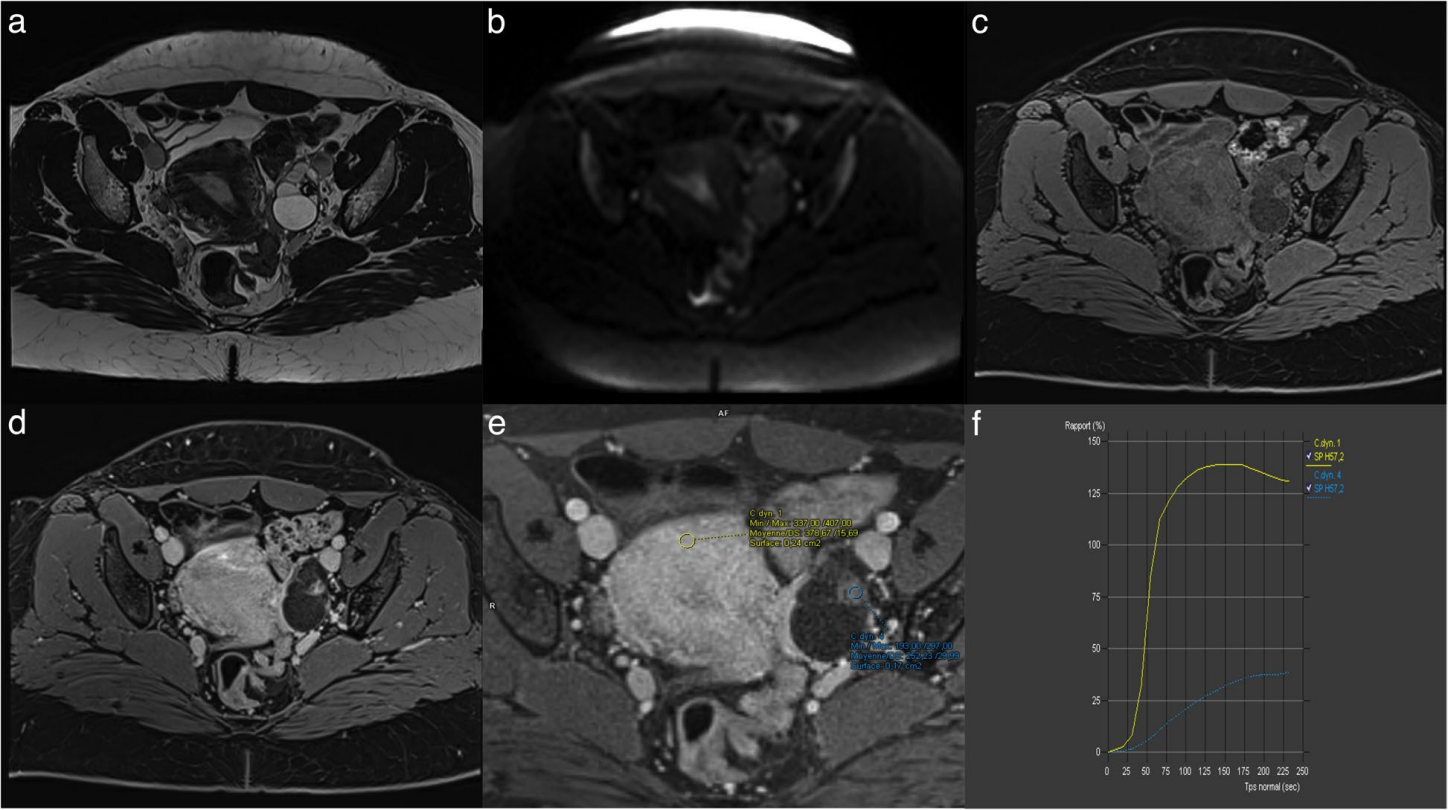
Example of left ovarian lesion with a solid component with a type I dynamic enhancement curve. a Axial T2-weighted sequence without fat saturation. b Axial DWI scan (*b*: 1200 s/mm^2^). c Axial unenhanced T1-weighted water sequence. d Axial late-gadolinium T1-weighted sequence. e, f DCE MRI T1-weighted sequence with corresponding curve of the tissular component showing a type I curve. This was favored to be a benign cystadenofibroma
